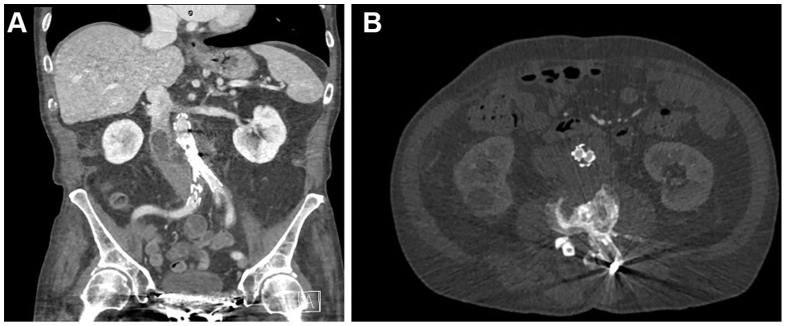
Computed tomography aortogram (CTA) on re-presentation. (A) Coronal demonstrating inferior vena cava (IVC) thrombus. (B) Axial.
